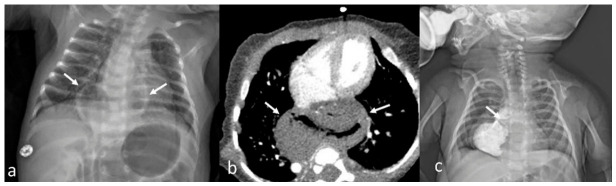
Radiological findings of hiatal hernia at 1 month of age. Posteroanterior chest radiograph demonstrates radiolucent gastric air located superior to the diaphragm and posterior to the heart (a). Axial computed tomography image shows findings consistent with hiatal hernia (b). Fluoroscopic examination shows the esophagogastric junction at the T7–T8 level, slightly to the right of the midline, with the gastric cardia and fundus positioned above the diaphragm, consistent with a type III hiatal hernia (c).
